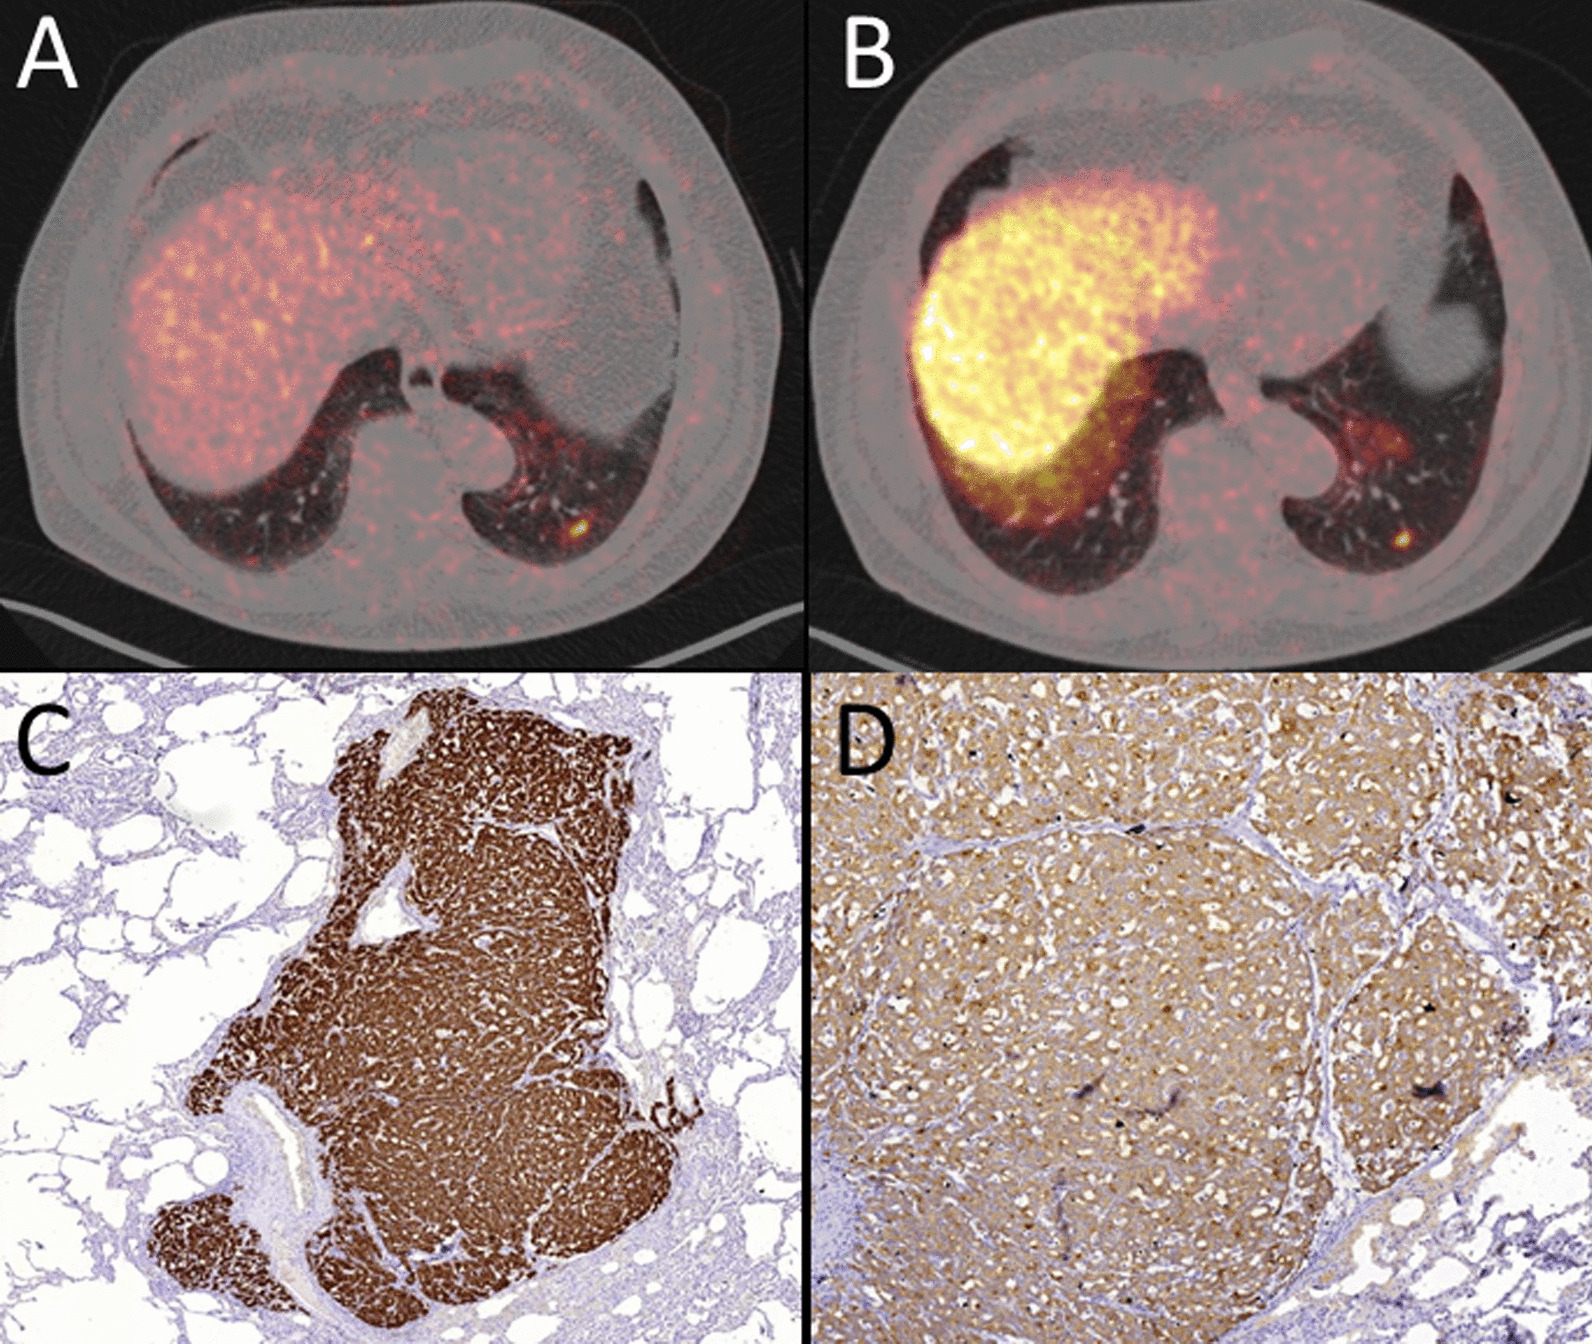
Axial fused [^68^Ga]-Ga-PSMA-11 PET/CT (A) and [^18^F]-F-PSMA-1007 (B) with concordantly intense focal uptake in a pulmonary metastasis in the left lower pulmonary lobe. Immunhistochemial confirmation of PSMA- (C) and PSA- (D) expression of the pulmonary metastasis (D)
